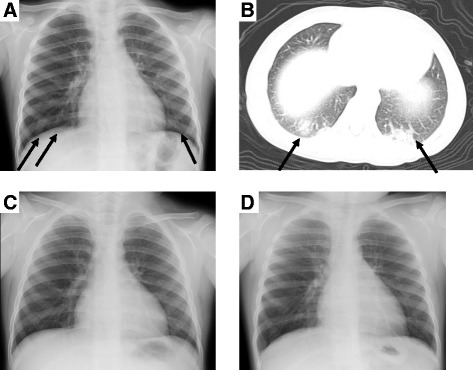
Chest radiograph (a) and computed tomography (b) show focal IP in the lower lobes during the acute phase, and no abnormalities at 4 months (c) and 8 months after the convalescent phase of JDM-MAS and IP (d). *Arrow* marks indicate focal IP
